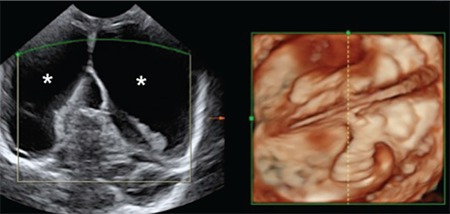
Postnatal transfontanellar ultrasound performed with three-dimensional volume reconstruction showing ventricular dilatation (*)
